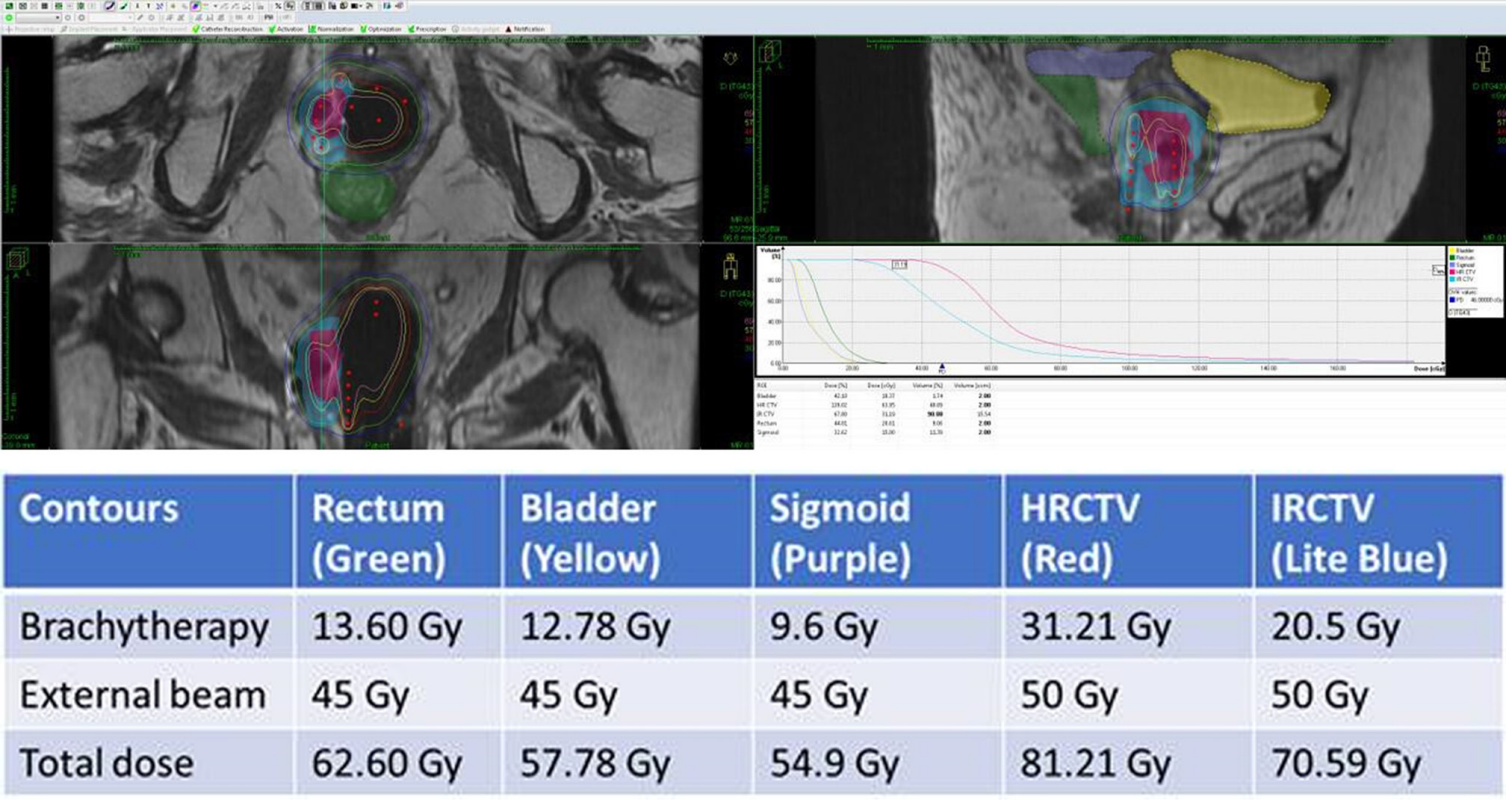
This figure shows an implant of a patient with right side vaginal tumor. The patient still had residual disease after external beam and therefore a multi-channel cylinder as well as free-hand interstitial needs were used. The needles were placed into the right vaginal wall through the perineum using ultrasound guidance. The planning was done using MRI and CT scan.
